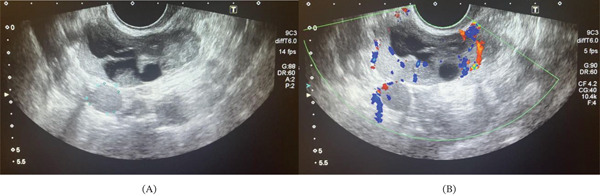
Ultrasound appearance of neoformation located in prevescical peritoneum (abdominal pregnancy) on (A) greyscale and (B) power Doppler imaging.
